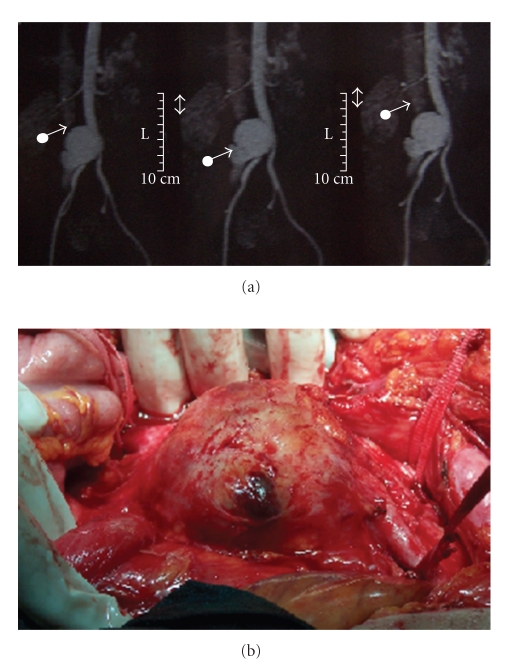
(a) MRI of abdominal aortic pseudoaneurysm showing a small amount of aortacaval connection. (b) Operative image of pseudoaneurysm.
